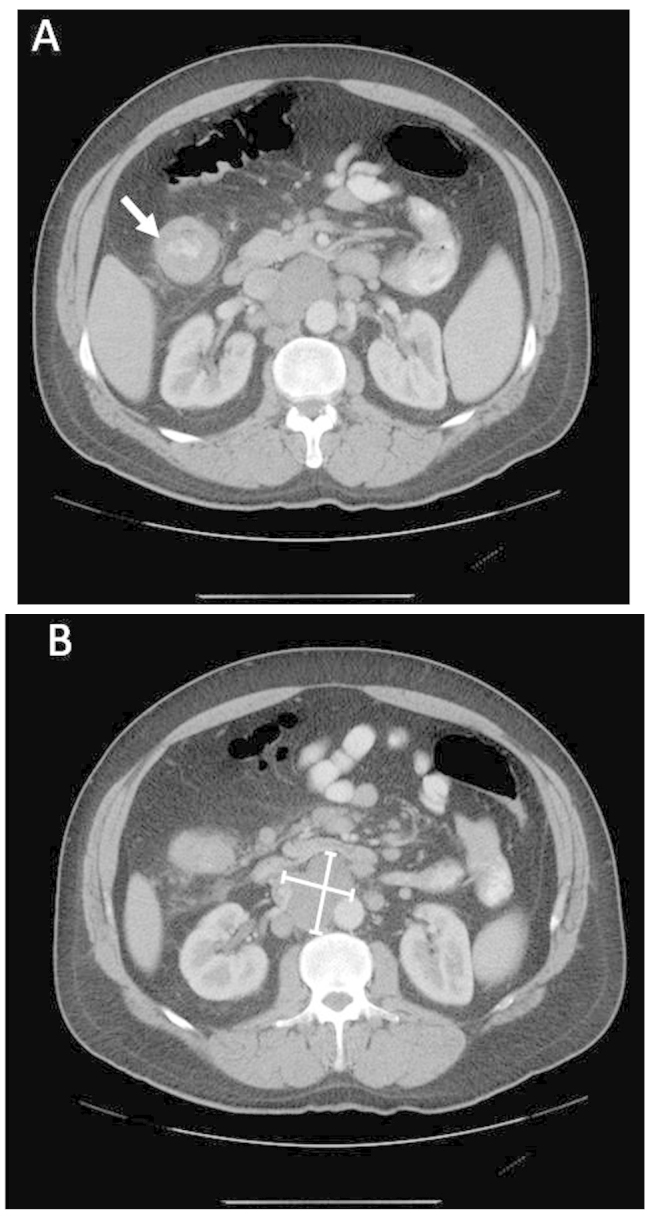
Computed tomography scan of the abdomen showing (A) the proximal colon mass and (B) the large peri-aortic lymph nodes.
